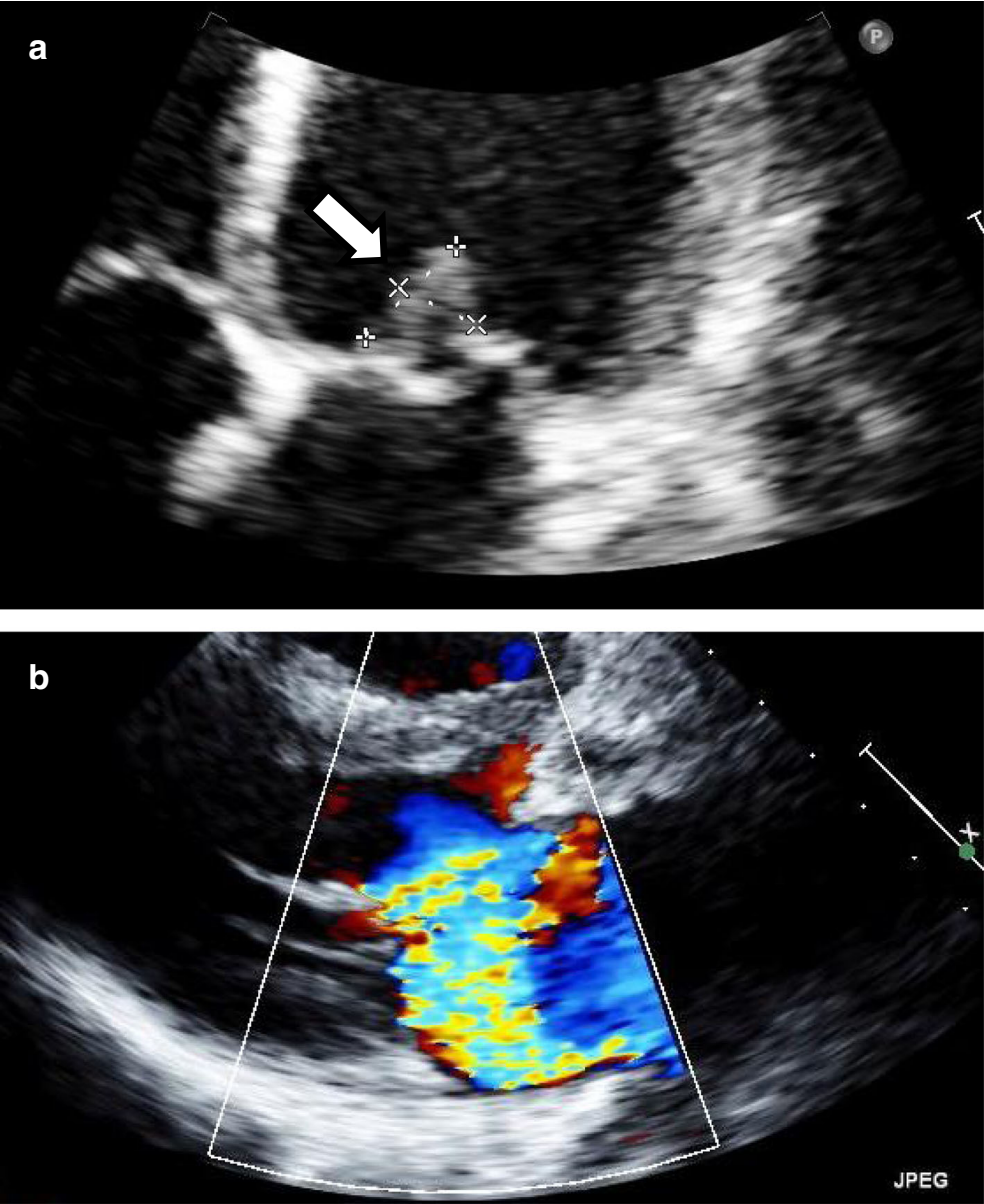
Echocardiogram. a. vegetation on mitral valve (arrow). b. moderate mitral regurgitation
